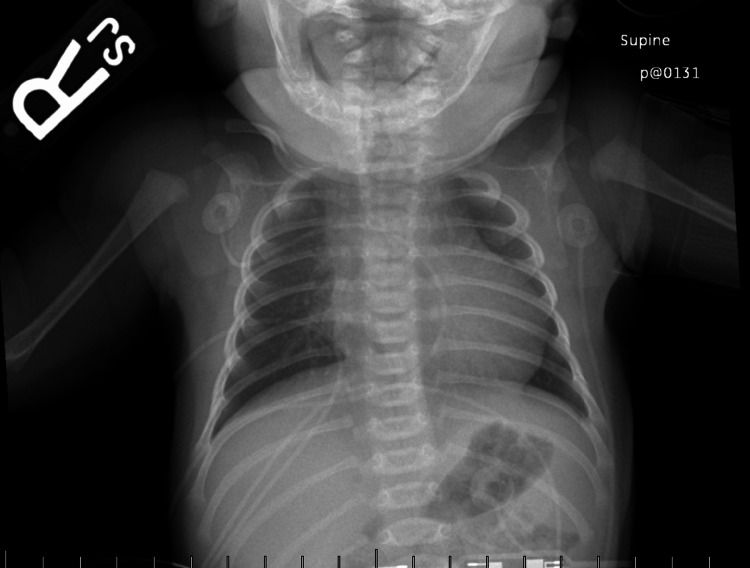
Chest X-ray of the patient upon admission into the CVICU at one day of age. Chest X-ray displays mild cardiomegaly with prominent and granular lung interstitium. CVICU: cardiovascular intensive care unit
